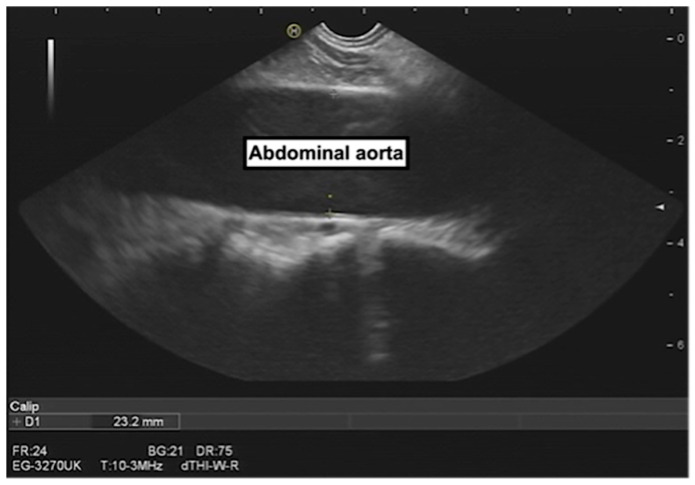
Ultrasonographic ultrasound demonstrated the anatomy of abdominal aorta.
